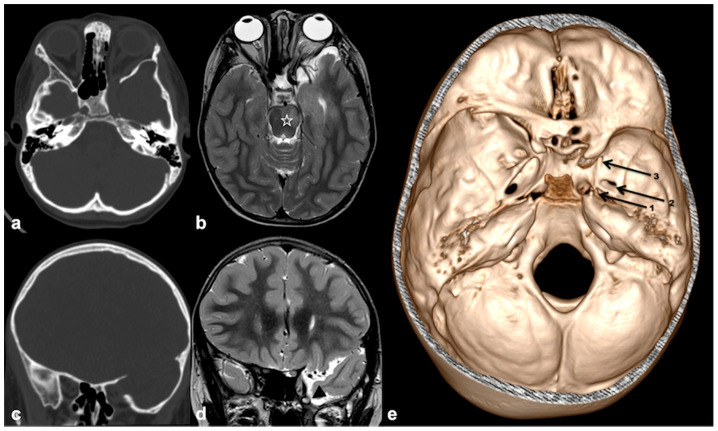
Axial CT (a) and TSE T2w MRI (b), and coronal CT (c) and TSE T2w MRI (d) of a 6 years-old boy with NF1, showing left sphenoid wing dysplasia with enlargement of the left orbit (bare orbit sign), bony defect of the posterior orbital wall and enlargement of middle cranial fossa (with associated meningocele—black arrowhead). Brainstem FASIs are also visible on axial TSE T2w MRI (white star). CT volume rendering (e) confirmed middle cranial fossa asymmetry with reduced foramina diameter on the affected side (black arrows: 1-carotid canal; 2-foramen ovale; 3-foramen rotundum).
